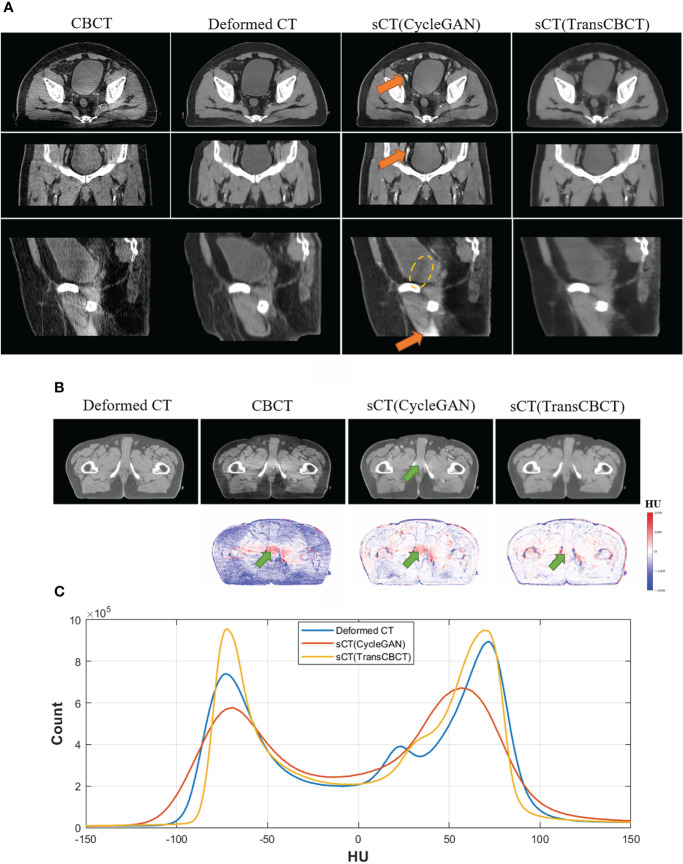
(A) Axial, sagittal, and coronal views of one patient. The orange arrows indicate the abnormal structure on sCT images. Yellow lines indicate the obvious beam hardening artifacts. Anatomical differences can be obtained, especially on the bladder sizes and locations, because of the time intervals between CT and CBCT scanning. The TransCBCT and CycleGAN maintained the same structure as CBCT with a clear organ edge. (B) Example of an axial slice of the deformed CT, CBCT, and sCT with the HU difference compared to the deformed CT image. The green arrows indicate the “photon starving” artifacts. (C) The HU histogram plot calculated on the entire test set.
